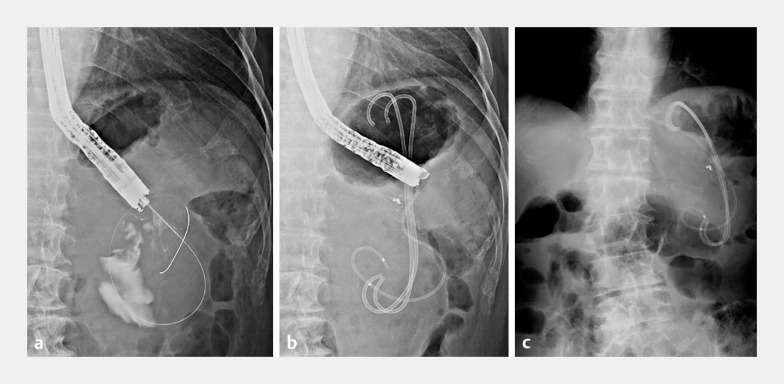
Endoscopic ultrasound-guided cyst drainage was performed for an infected hematoma with insertion of two plastic stents and a nasal drainage catheter.
